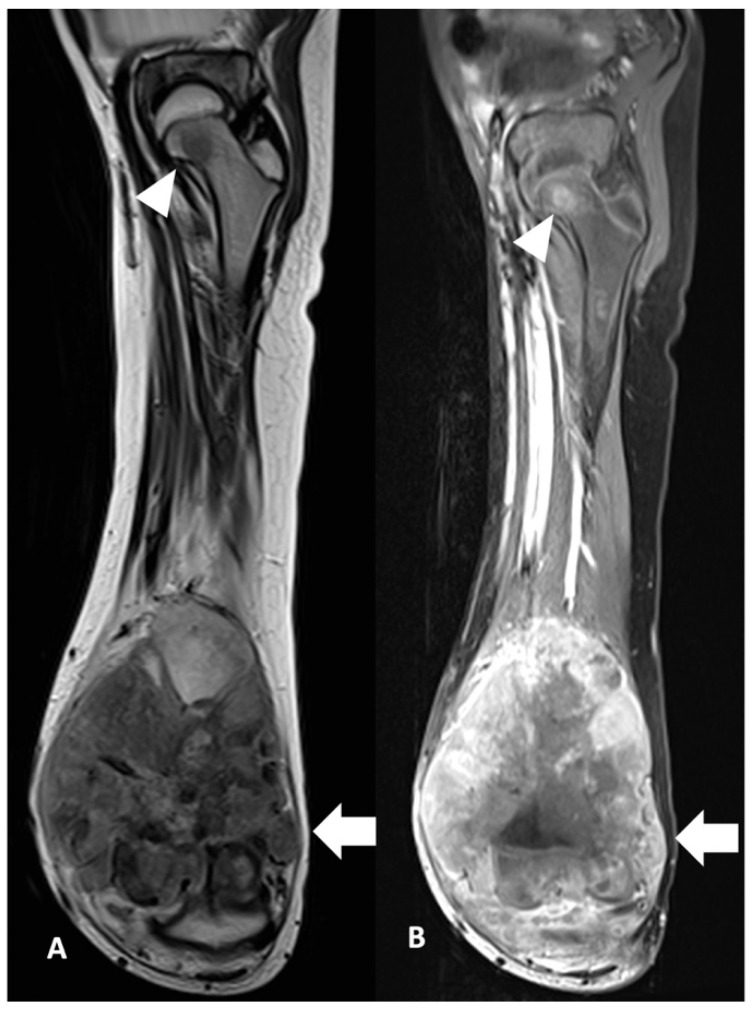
Skip metastasis. Coronal view of MRI left femur of a 10-year-old boy in (A) T2W and (B) T1FS CE show distal osteosarcoma (white arrow) with skip metastasis at the femoral neck (white arrowhead). The child had tumour progression post-treatment within 2 years of diagnosis.
